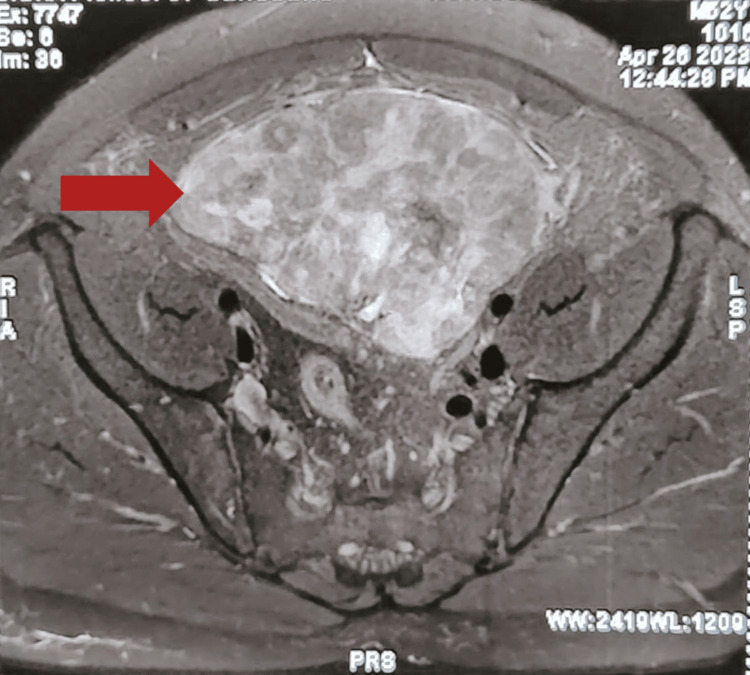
Transverse section of MRI abdominal scan, showing the tumor (red arrow) arising posterior to the anterior abdominal wall without infiltrating the urinary bladder MRI: magnetic resonance imaging
